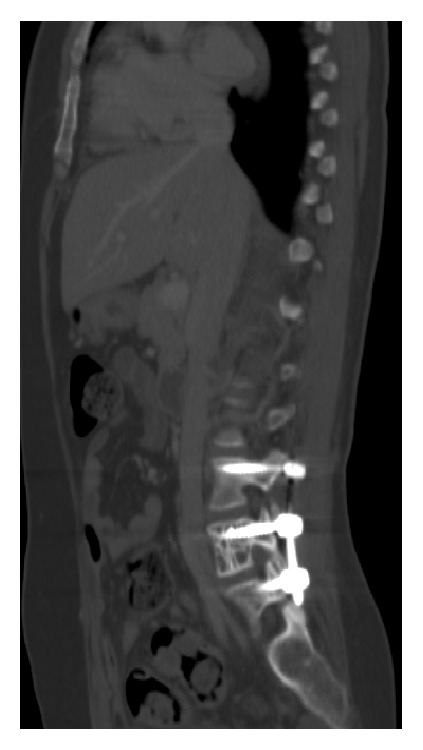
CT. Sagittal view. Pedicle screw fixation of L3-L5 after subtotal tumorectomy.
